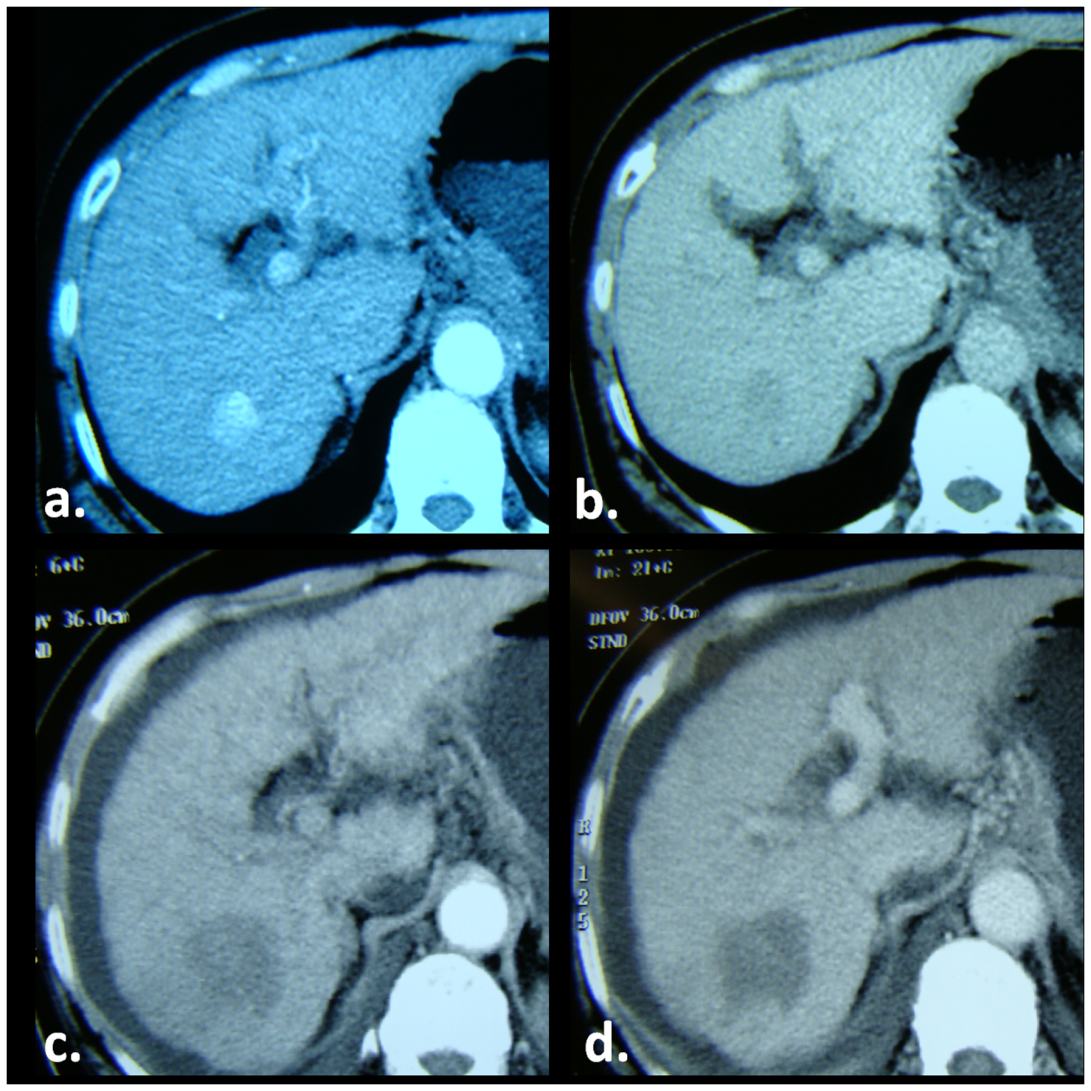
MW ablation of a HCC in a 50-year-old man with hepatitis B–related liver cirrhosis. (a) Arterial and (b) portal venous phase pretreatment CT images show tumor as a small 22-mm intense arterial enhancement nodule on a, with contrast washout on b. (c) Arterial and (d) portal venous phase CT images obtained 1 month after treatment show tumor has been replaced by nonenhancment ablation zone with a diameter greater than that of treated tumor. Findings are consistent with complete ablation (CA).
